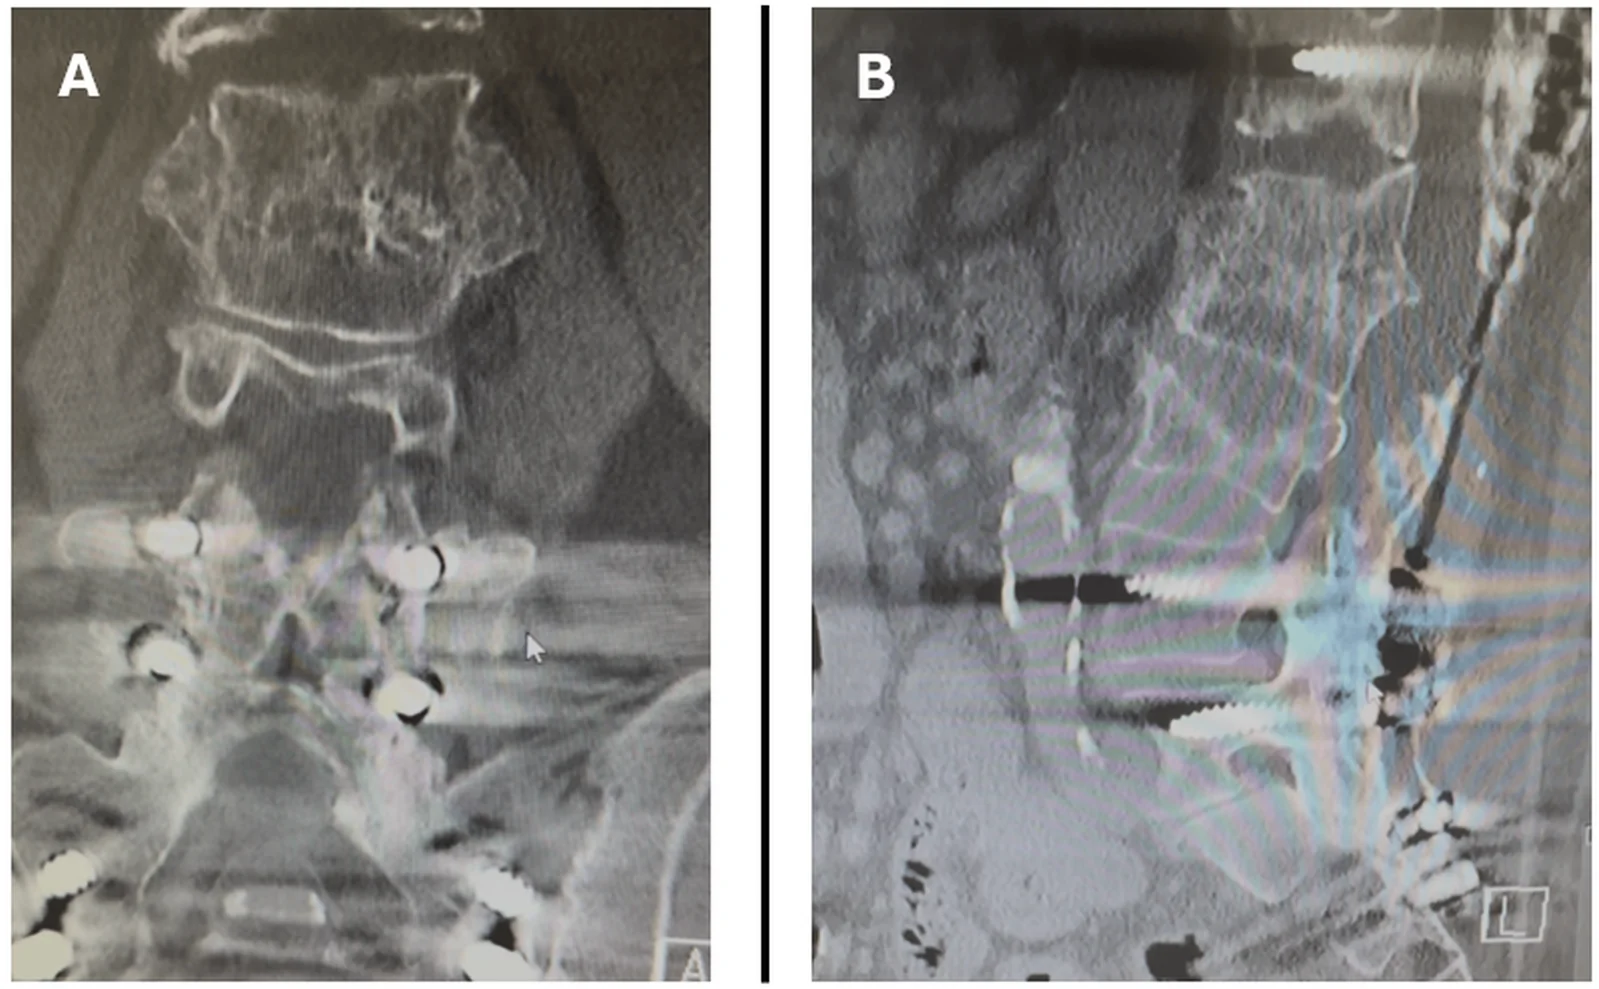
Postoperative CT imaging at eight months demonstrating bridging bone and early anterior column consolidation through the L1-L2 defect. (A) Coronal and (B) sagittal reconstructions show consolidated bone graft through the transpedicularly packed void in the absence of any anterior cage.
